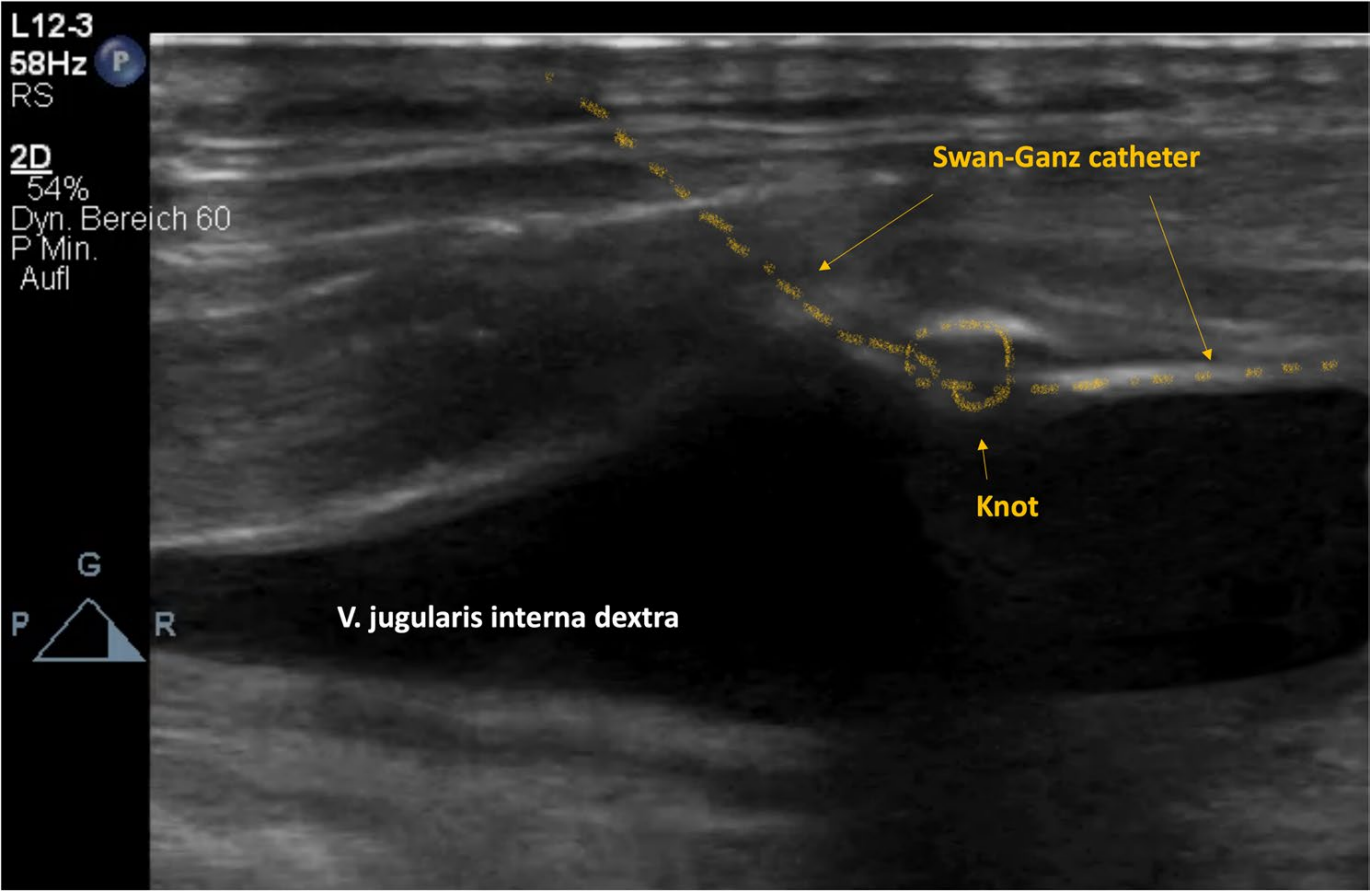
Ultrasound examination of the jugular vein
